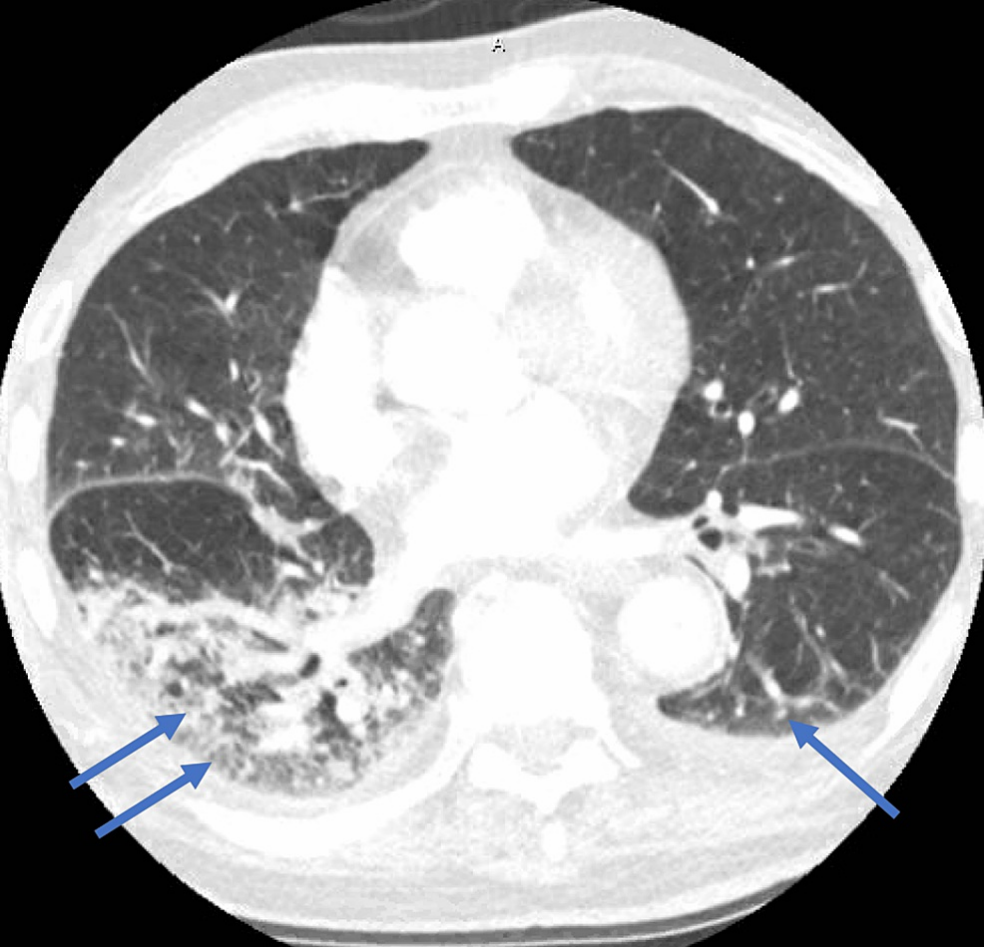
CT angiography of the chest showing acute pulmonary infiltrates in lower lobes
